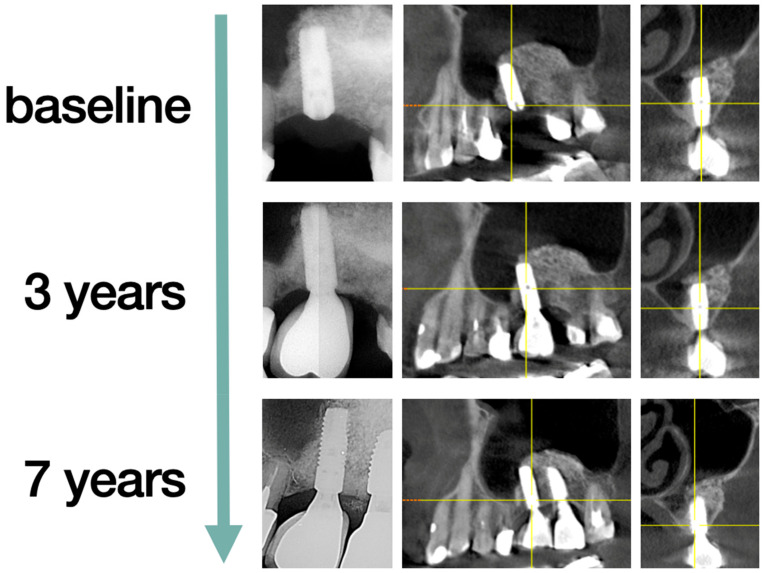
Regular implants. The same patient from baseline to seven years, implant tooth 26, follow-up. The second implant was placed after tooth 27 was lost and restored with a second single implant crown and is not included in the measurements. Periapical X-ray and CBCT are presented.
